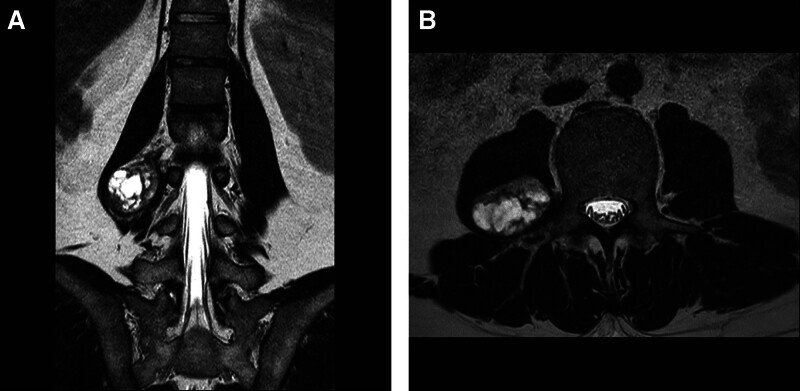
Preoperative magnetic resonance imaging (MRI) findings. A well-demarcated mass lesion in the right psoas muscle was identified in the (A) coronal and (B) axial views of MRI.
